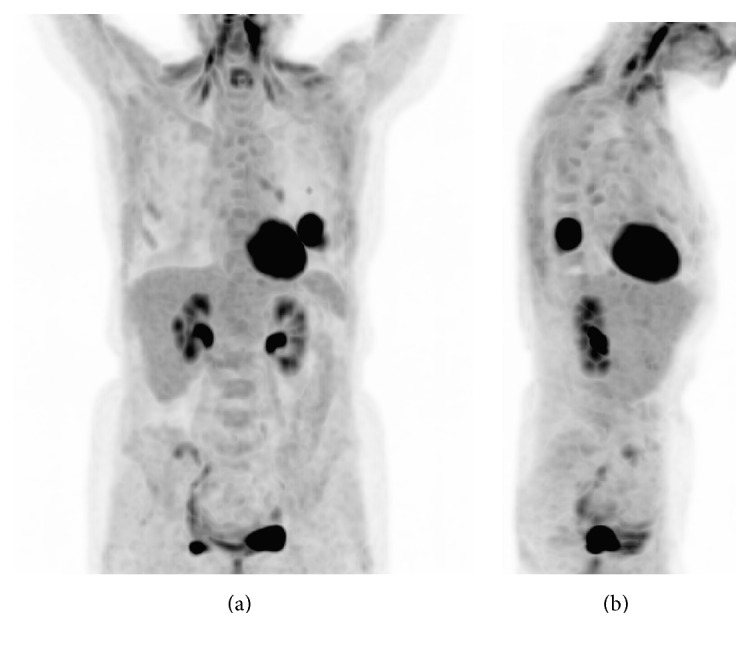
Coronal and lateral maximum intensity projection (MIP) images of the PET showing intensely FDG avid left lung mass and associated left hilar lymphadenopathy.
